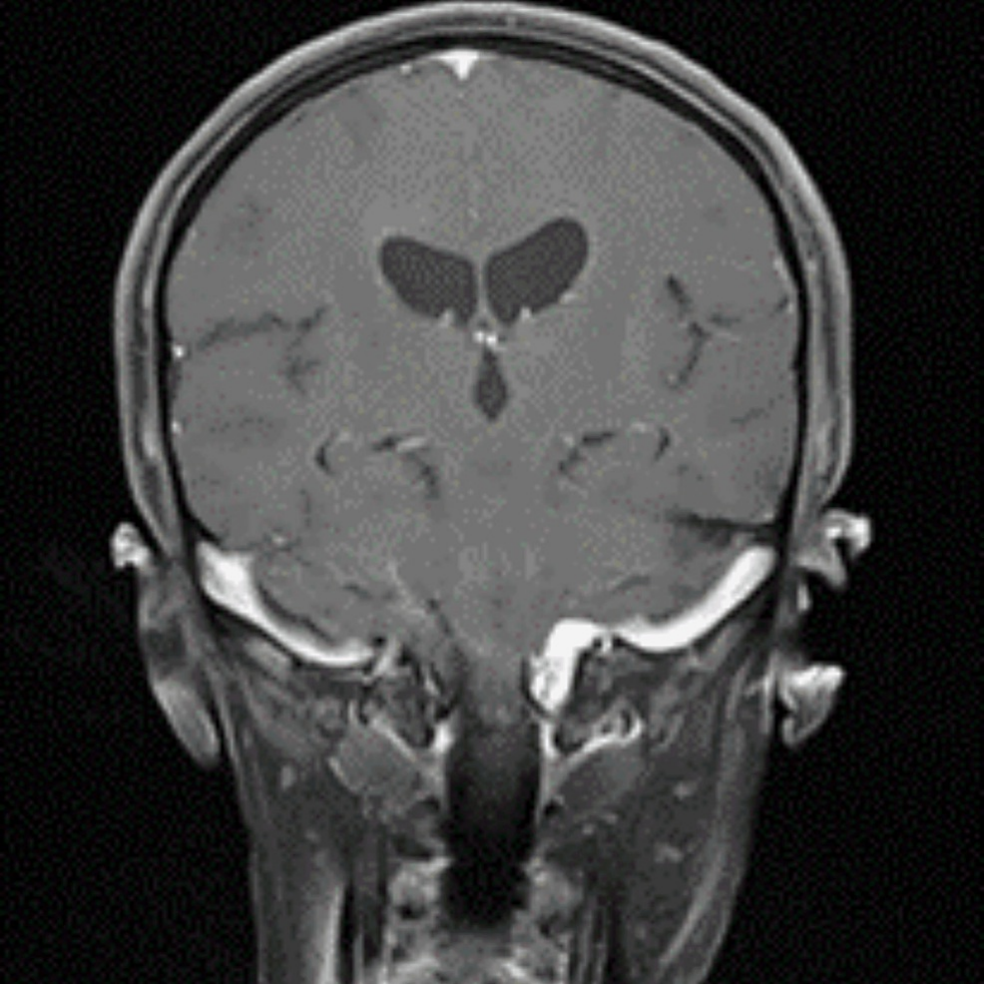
Post-operative cranioencephalic MRI. MRI: magnetic resonance imaging.
